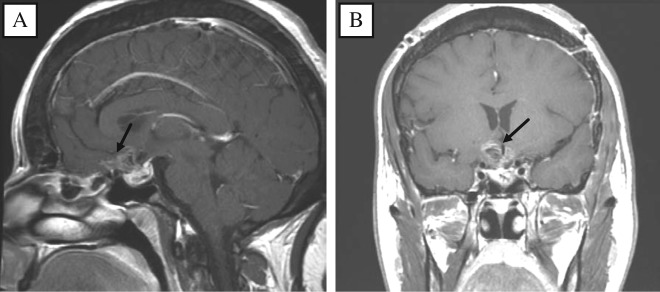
Sagittal (A) and coronal (B) T1 gadolinium-enhanced MRI postoperative images showing enhancing residual tumour (black arrows).
